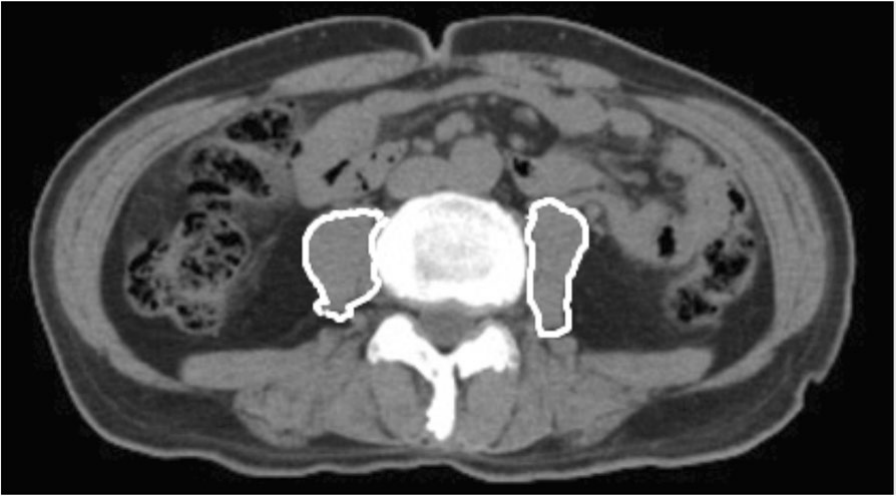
Cross-sectional computed tomography image at the third lumbar vertebral level.The areas of the bilateral psoas muscle were measured by manual tracing
